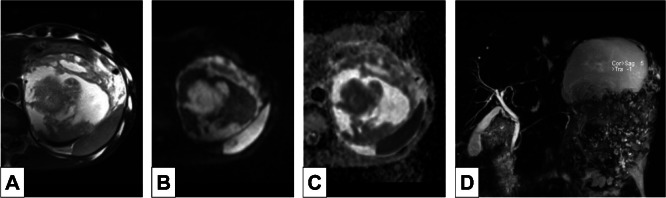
MRI. (A) T2-weighted image (T2WI). (B) Diffusion-weighted image (DWI) (b = 800). (C) Apparent diffusion coefficient (ADC) map. (D) Magnetic resonance cholangiopancreatography (MRCP). The hyper intense portions of the tumor on T2WI show no diffusion restriction and also appear hyper intense on MRCP. The hypointense portions of the tumor on T2WI show diffusion restriction. No dilatation of the main pancreatic duct is seen on MRCP.
